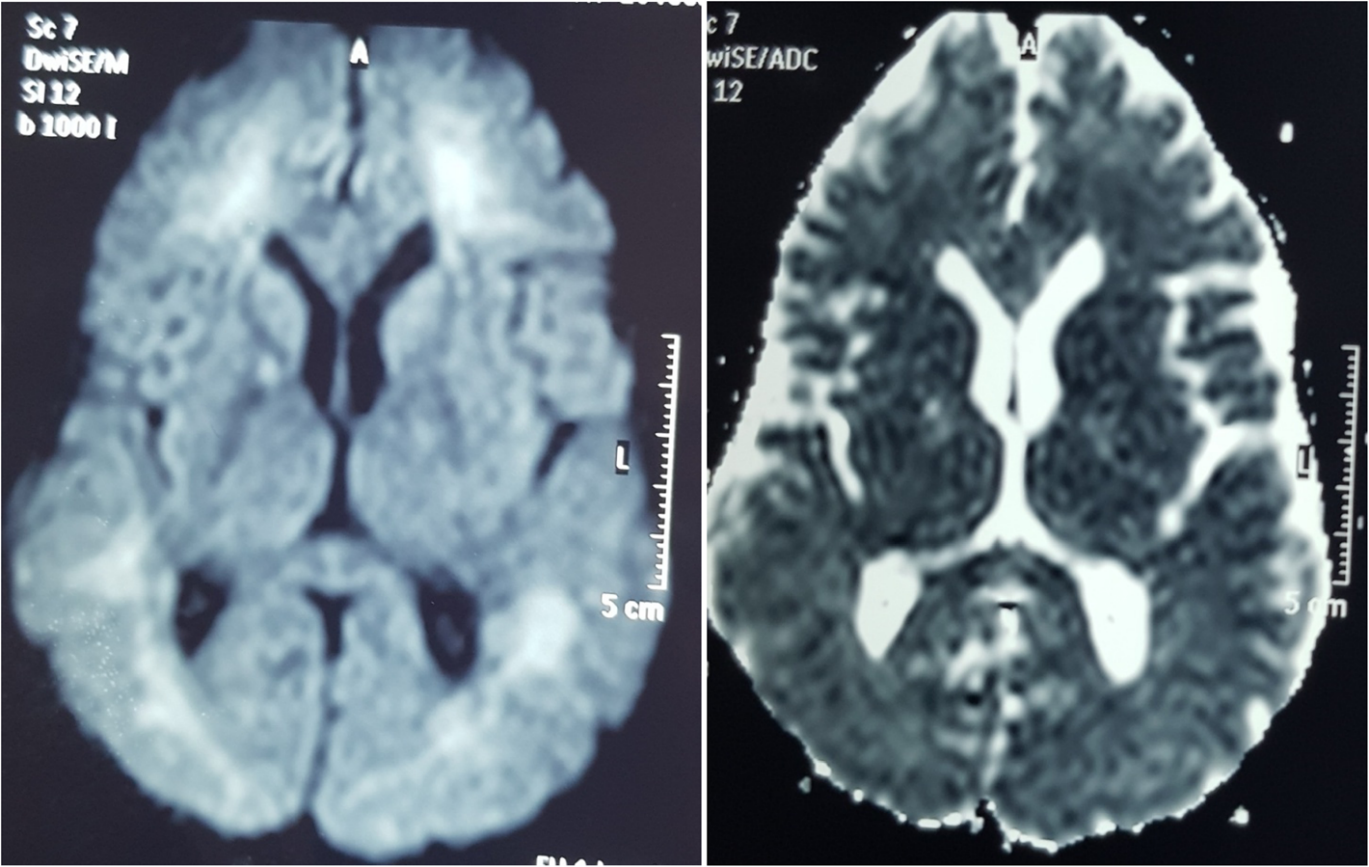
MRI brain showing high signals in DWI images with no significant changes appreciated on ADC map in subcortical white matter, globus pallidus
